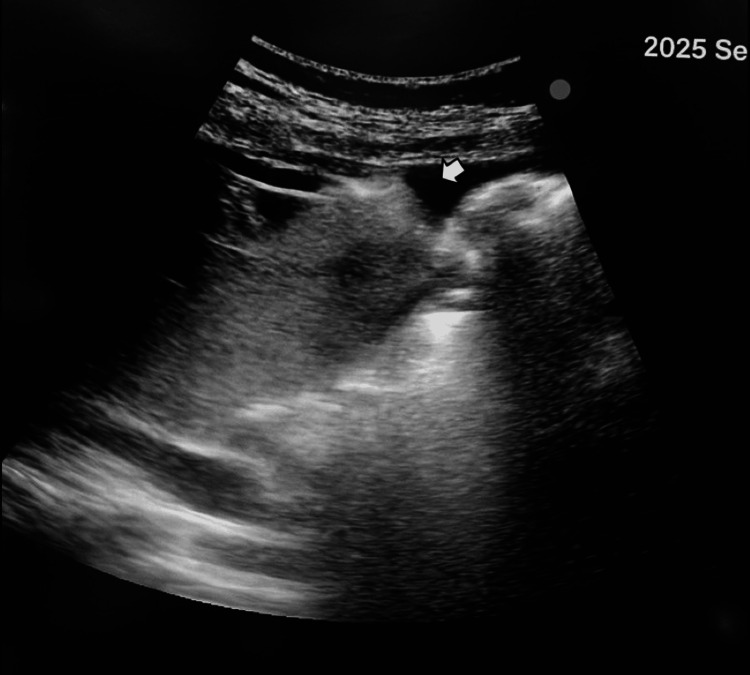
Ultrasound image demonstrating moderate anechoic peritoneal effusion (white arrow) in the hepatorenal recess, consistent with reactive or hemorrhagic ascites.
